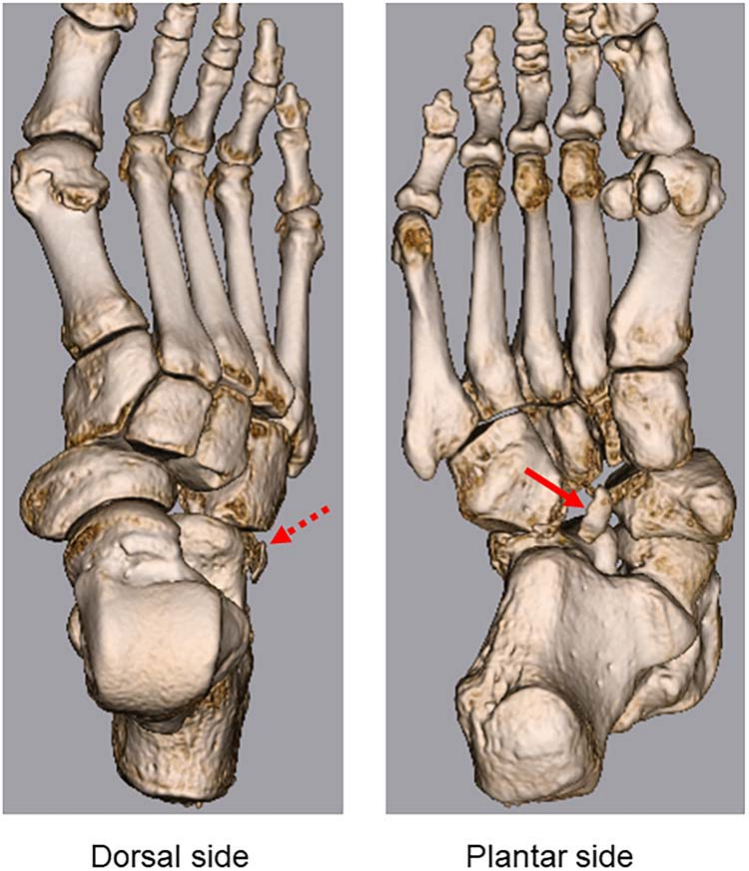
3D CT shows lateral dislocation of the naviculocuneiform and calcaneocuboid joints of the right foot, with fractures of the anterior edge of the calcaneus (broken arrow) and medial edge of the cuboid (arrow).
